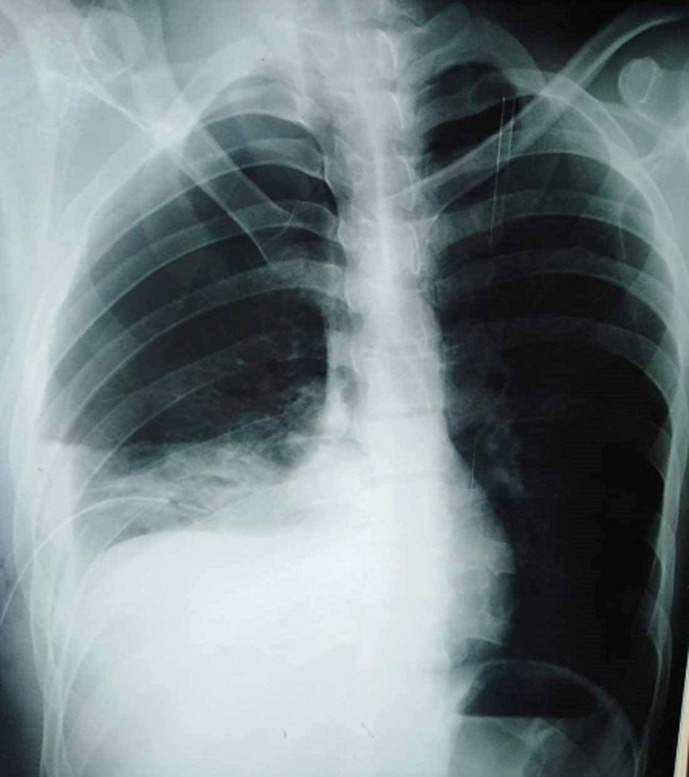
control chest X-ray showing recurrence and increasing of the right pleural effusion and chest drainage in place
